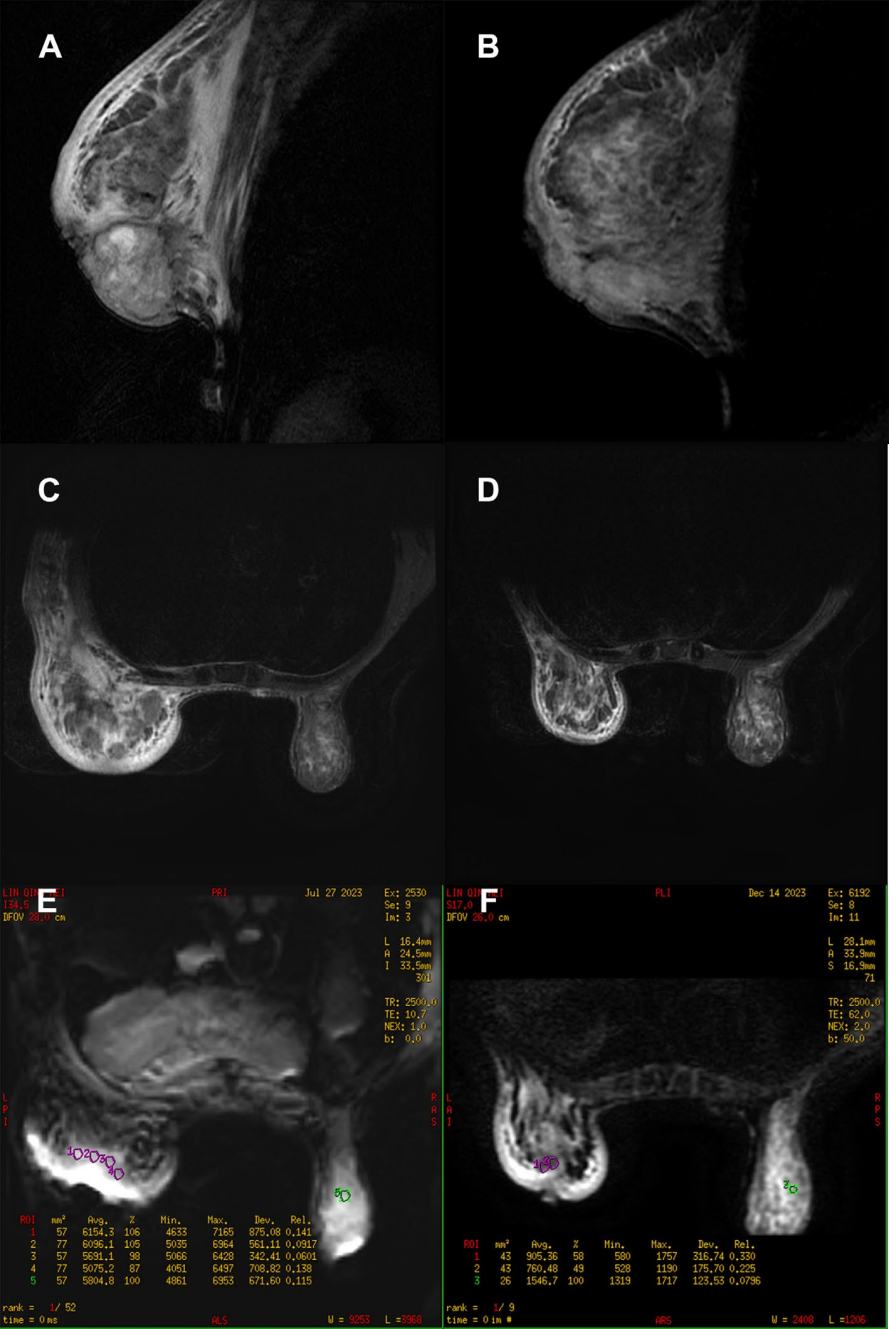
Comparison of breast MRI before and after six cycles of neoadjuvant therapy. The left (A, C, E) is the breast MRI image before neoadjuvant treatment, and the right (B, D, F) is the breast MRI image after neoadjuvant treatment.
